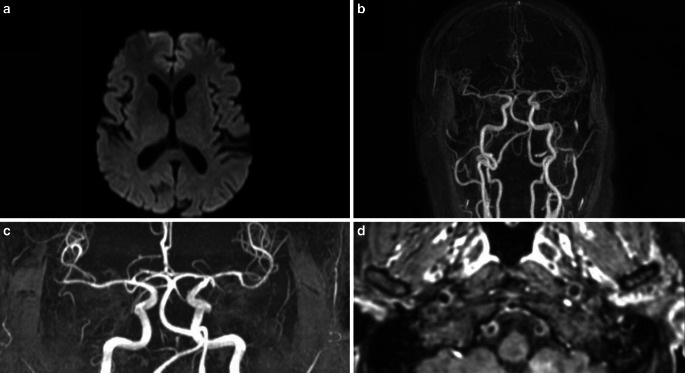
A 61-year-old patient hospitalized for dyspnea due to SARS-CoV‑2 infection with intubation over 10 days. His medical history included ischemic heart disease and sleep apnea syndrome, but no psychiatric or cognitive impairment. He had a persistent and unexplained delirium persisting for 12 days after stopping all sedative drugs, without neurological deficit. Magnetic resonance imaging: no changes on DWI (a). Absence of stenosis on CE-MRA (b) and TOF MRA (c) Bilateral enhancement of the vertebral arteries on black blood VISTA images (TE: 17 ms, TR: 400 ms, image thickness 1.5 mm) (d)
